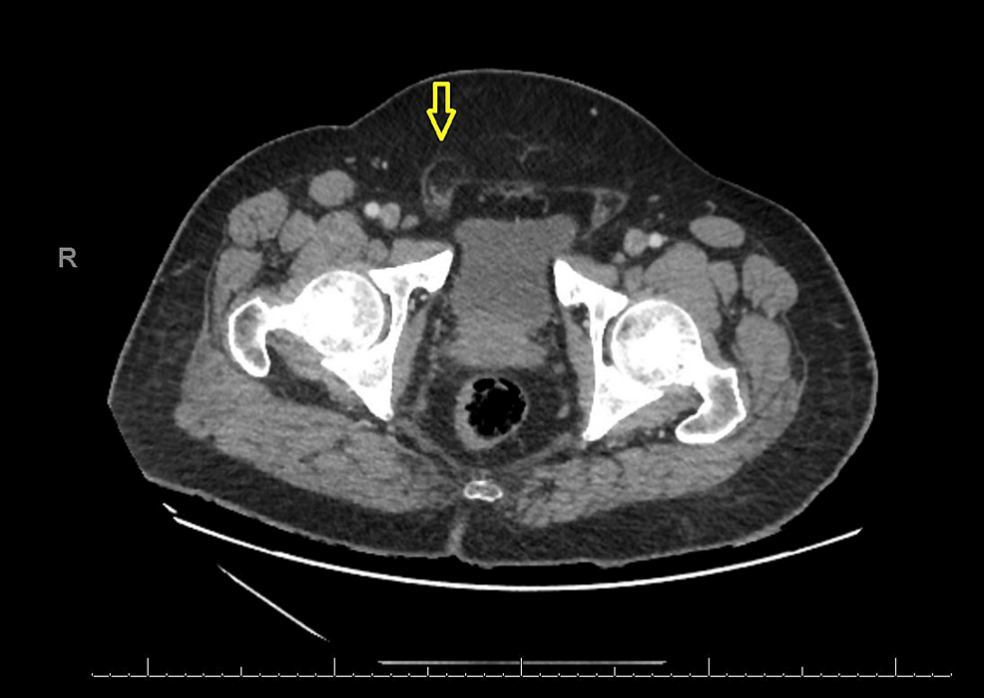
A CT scan shows prominent fat in the proximal part of the right inguinal canal, which suggests the presence of a partially patent processus vaginalis.
